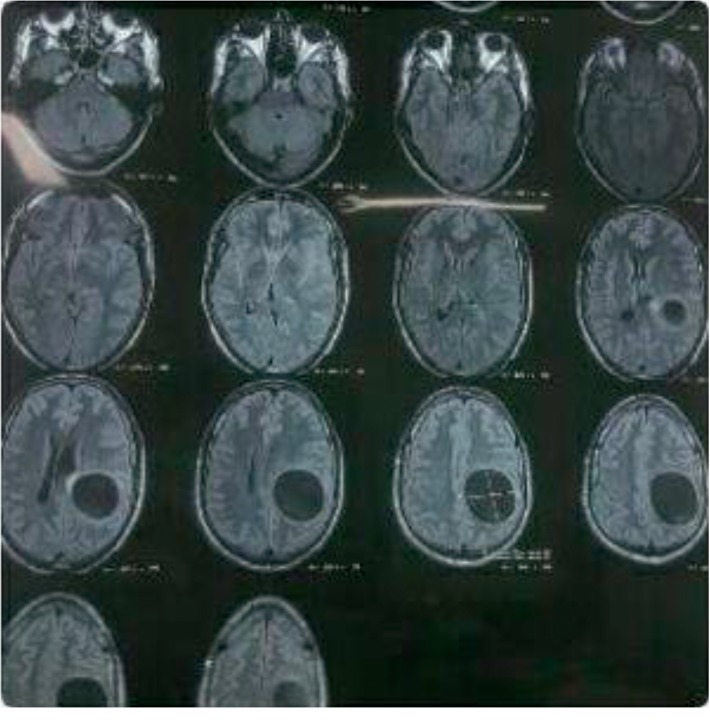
CT scan showing a large cyst affecting the left fronto-parieto-occipital region.
